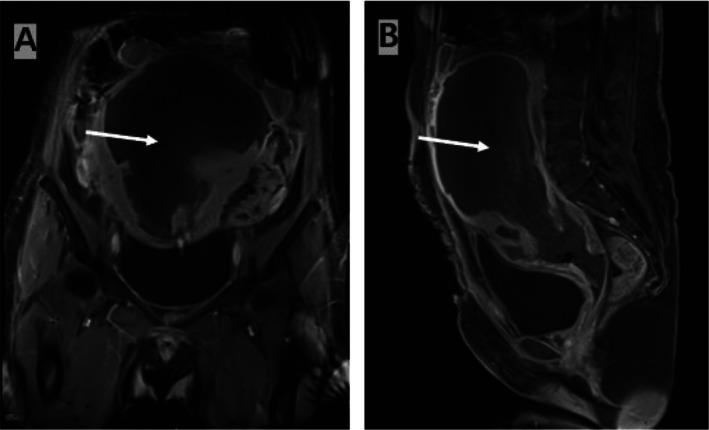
Pelvic enhanced MRI, arrows showing unenhanced necrotic myometrium: (A) coronal position; (B) sagittal position.
